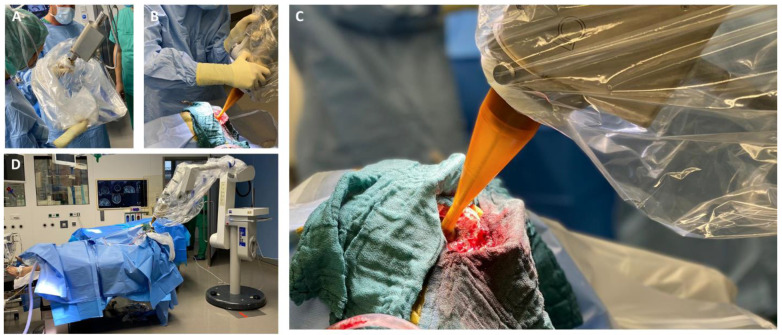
(A) Connection of the spherical applicator with the X-ray source and sterile draping; (B) Manual applicator positioning into the resection cavity; (C) Intraoperative view of the inserted applicator in the resection cavity; (D) Intraoperative set-up prior to the start of IORT.
